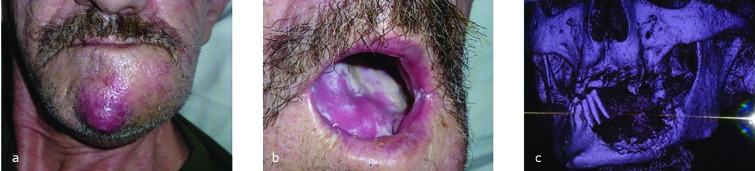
a, b, c: Clinical appearance and dental CT of the patient
